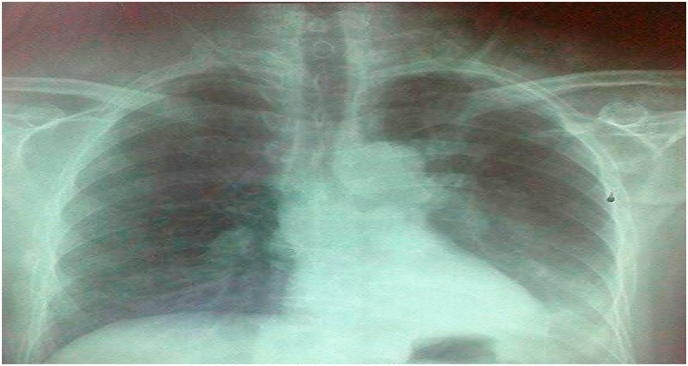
X-ray of chest face revealed opacity at the level of the left middle arch with opening of the angle of the keel.
